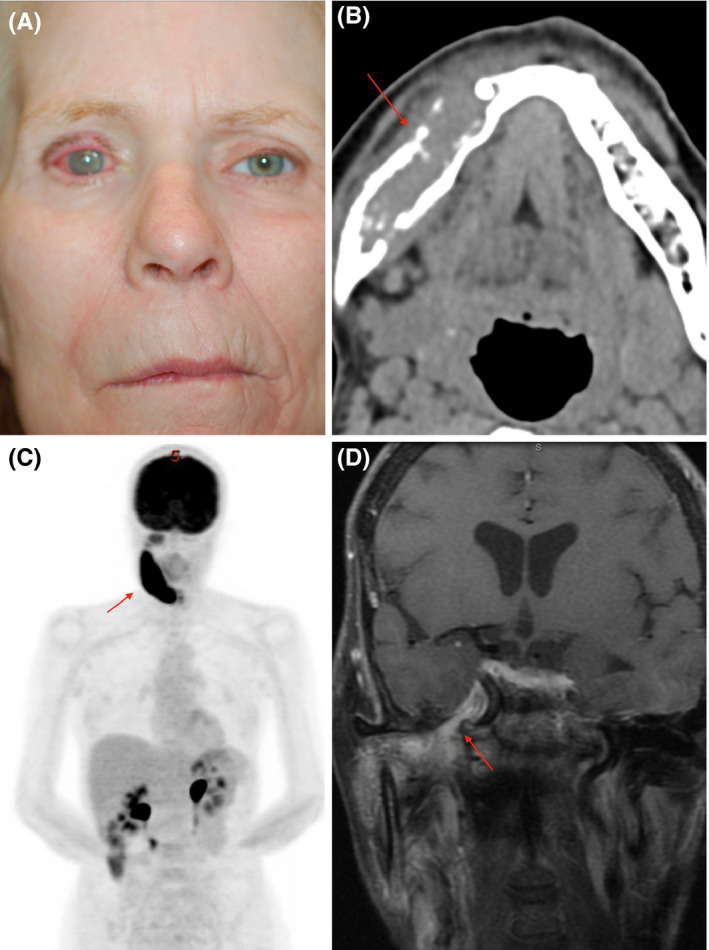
A, External photograph showing right keratopathy, facial nerve paralysis, and temporal wasting (B). Axial computed tomography (CT) demonstrating an osseous lesion of the right mandible (red arrow). C, Fluorodeoxyglucose (FDG)‐positron emission tomography (PET) with FDG uptake of the right mandible (red arrow). D, MR coronal T1 FSGD with enhancement of the trigeminal nerve (red arrow)
